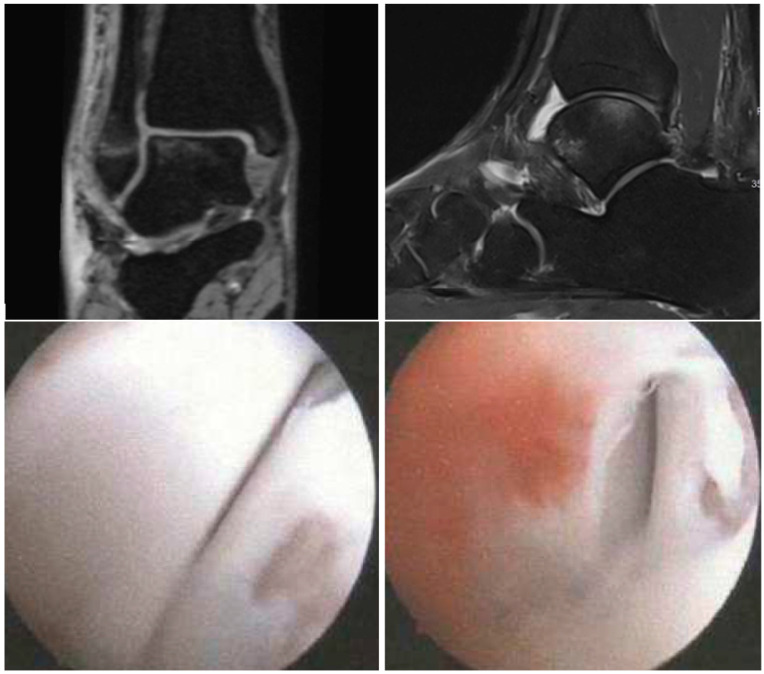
Coronal (above left) T2 3D-double-echo with water excitation (T2 DE3D WE) and sagittal (above right) proton-density-weighted turbo-spin-echo fat-saturation (PD tse fs) MRI sequences showing a 3 × 3 mm ICRS 3a CL in zone 5 of the talar dome with subchondral edema in a 20-year-old female with an acute ankle fracture. Below are the intraoperative figures of the corresponding lesions in arthroscopy in zone 5 of the talar dome measuring 6 × 6 mm and grading ICRS 3b.
